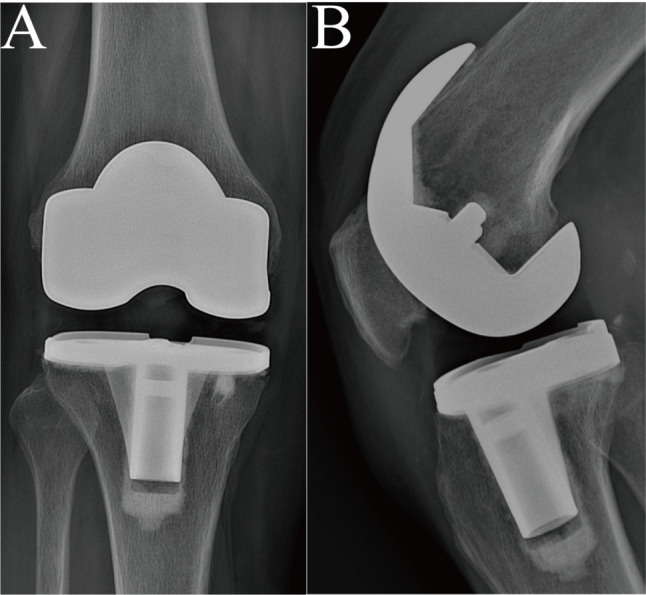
Twelve-month follow-up radiographs after revision total knee arthroplasty (TKA). A Anteroposterior view: The revision TKA components are well-positioned with satisfactory mechanical alignment. No radiolucent lines or loosening are observed at the prosthesis-bone interface. B Lateral view: The implant maintains appropriate sagittal alignment, with no evidence of component migration, wear, or periprosthetic lucency
